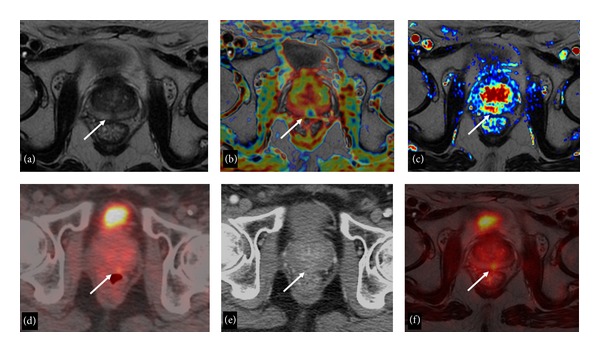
72-year-old man with biopsy-proven local PC recurrence after EBRT. T2-weighted axial image (a) demonstrates a hypointense focal area close to the midline (arrow). The ADC map (b) demonstrates that the nodular area (arrow) has significantly lower ADC values than the surrounding parenchyma. The wash-in perfusion map (c) shows a high wash-in rate (arrow). On the ^18^F-Choline-PET/CT axial image (d) a doubtful PET-positive focus (arrow) is appreciable, while the lesion is not detectable on CeCT axial image (e). Fused ^18^F-Choline-PET/MRI image (f) demonstrates precise correspondence between PET-positive focus and MRI finding (arrow).
